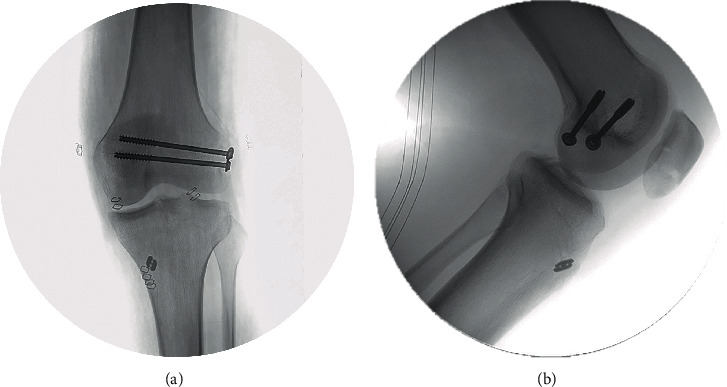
Coronal (a) and sagittal (b) intraoperative X-ray showing the final reduction and fixation of PCL and LCL avulsion fractures.
